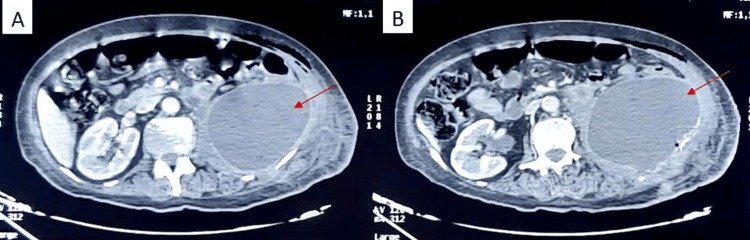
Axial CT images of the abdomen (A,B) showing a left retroperitoneal collection.
